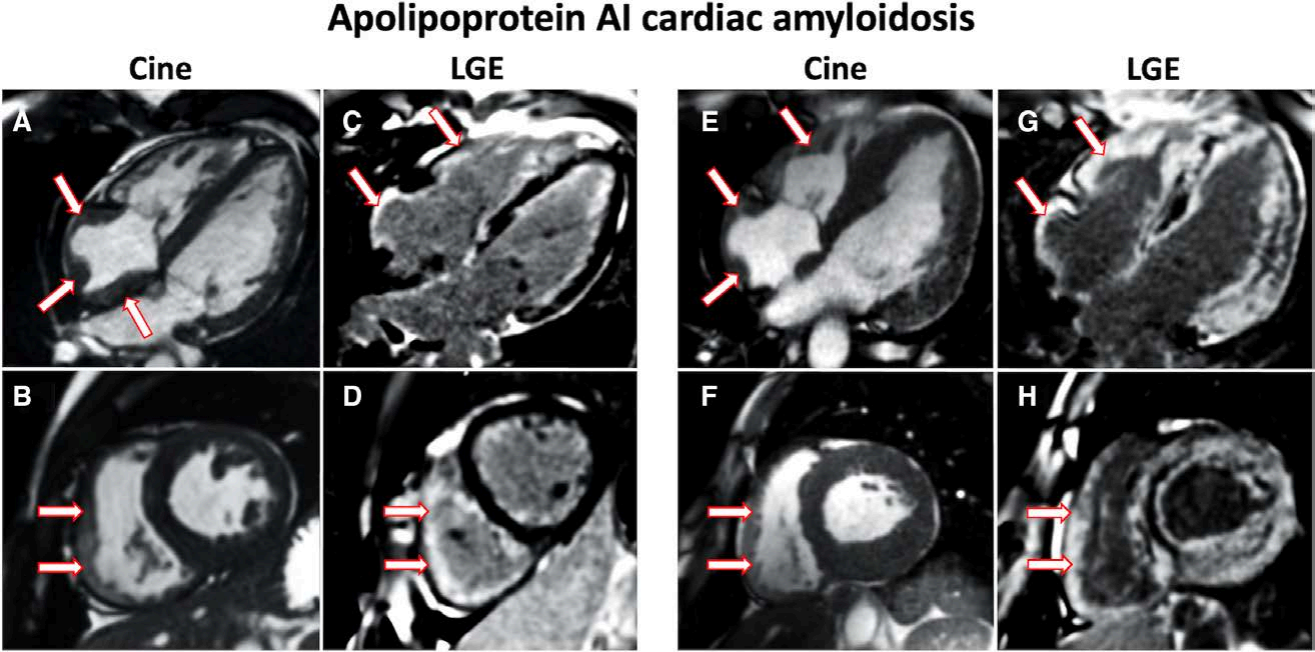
Cardiac magnetic resonance images of patients with Apo AI cardiac amyloidosis. A, 4-chamber cine image acquired with steady-state free precession sequence demonstrating prominent right atrial thickening (arrows). B, Short axis cine image demonstrating prominent right ventricular thickening (arrows). C, 4-chamber late gadolinium enhancement (LGE) image acquired using phase-sensitive inversion recovery sequence reconstructions with steady-state free precession read-outs, demonstrating prominent right atrial and right ventricular LGE (arrows). D, Short axis LGE image demonstrating prominent right ventricular LGE (arrows). E, 4-chamber cine image demonstrating prominent right atrial (arrows) and right ventricular thickening (arrows). F, Short axis cine image demonstrating prominent right ventricular thickening (arrows). G, 4-chamber LGE image demonstrating prominent right atrial and right ventricular LGE (arrows). H, Short axis LGE image demonstrating prominent right ventricular LGE (arrows).
